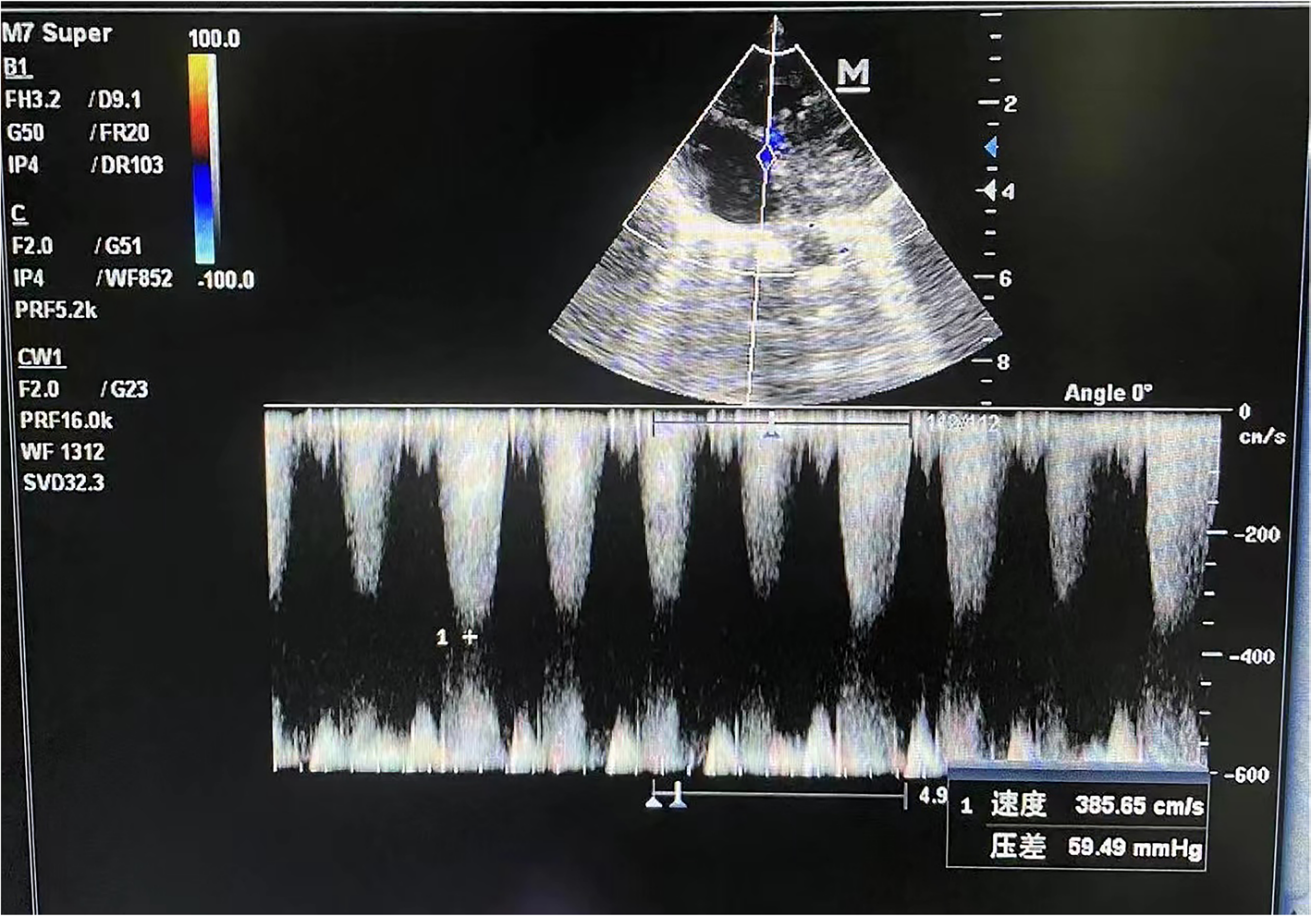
Echocardiogram showed tricuspid regurgitation with a peak jet velocity of 3.9 m/sec
